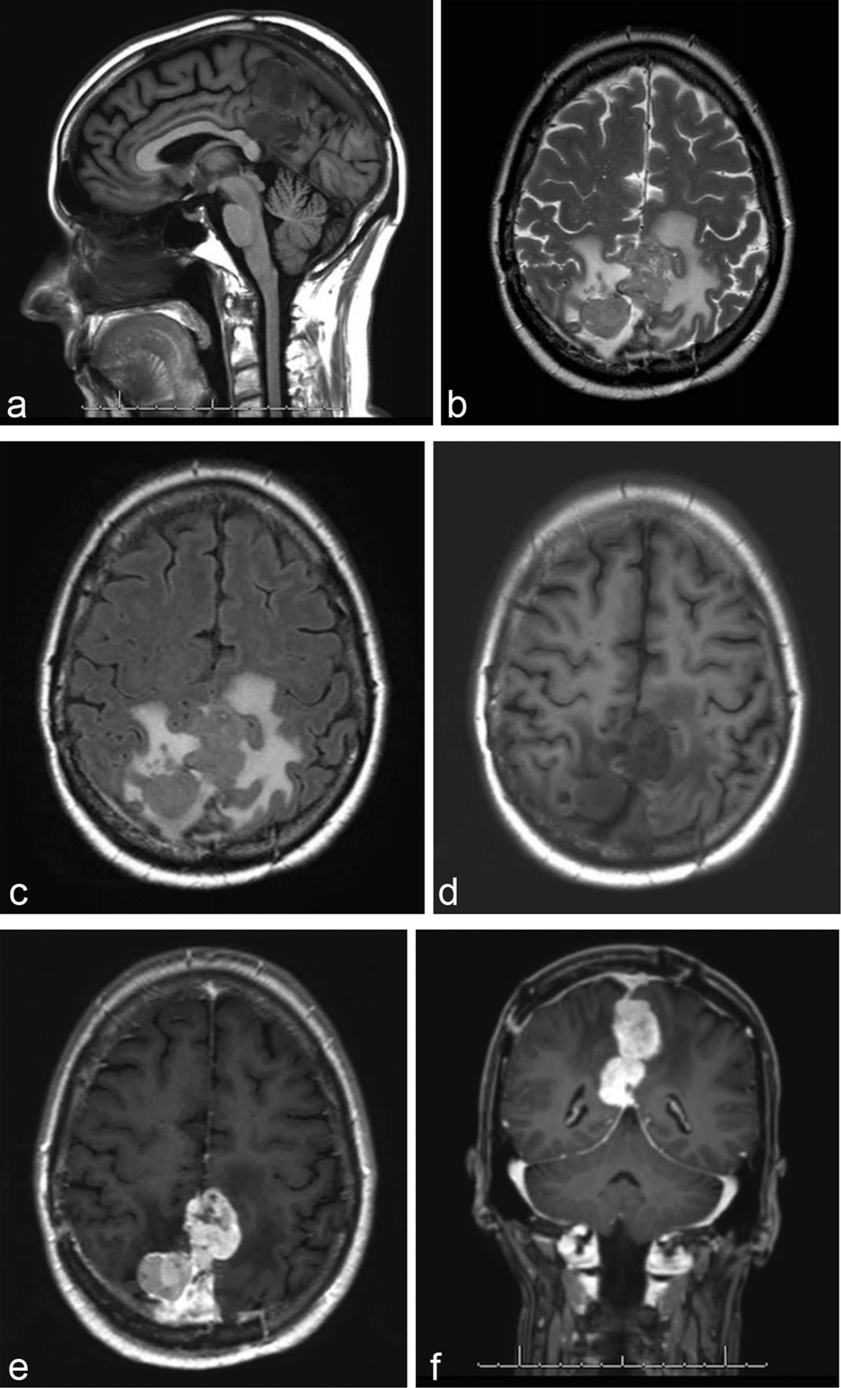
MRI with and without contrast of the brain, top row left to right sagittal *T*_1_, axial *T*_2_, fluid-attenuated inversion-recovery and bottom row left to right axial *T*_1_ precontrast, axial *T*_2_ postcontrast, coronal *T*_1_ postcontrast. MRI demonstrates recurrent multilobulated heterogeneous mass with associated oedema and avid enhancement. There is extension into the falx-tentorial region and likely involvement of the superior sagittal and straight sinuses. There is mass effect on the corpus callosum posteriorly.
